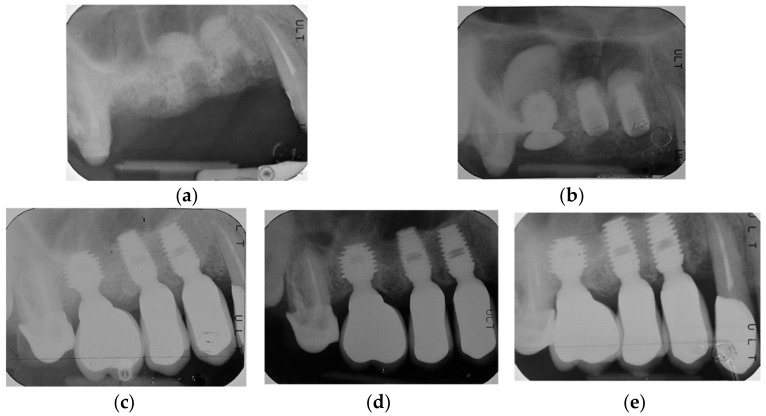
Single implants placed in 1.4, 1.5, and 1.6 sites (4.5 × 8 mm, 4.5 × 8 mm and 5 × 6 mm): (a) pre-operative radiograph before implant placement; (b) radiograph obtained at time of placement; see implant at 1.6 site with sinus lift temporary abutment designed to prevent displacement of the implant into the sinus, also see augmented sinus floor (1.4 sinus lift = 3.8 mm; 1.5 sinus lift = 2 mm; 1.6 sinus lift = 8.5 mm); (c) radiograph obtained at time of loading; (d) radiograph obtained at 3-year follow-up; (e) radiograph obtained at 5-year follow-up. See graft resorption after 3 years and stable bone levels with minimal changes between 3-year (1.4 RCBH = 7.5 mm; 1.4 sinus lift = 2.55 mm; 1.5 RCBH = 6.45 mm; 1.5 sinus lift = 0.5 mm; 1.6 RCBH = 7.1 mm; 1.6 sinus lift = 4.5 mm) and 5-year follow-up (1.4 RCBH = 7.12 mm; 1.4 sinus lift = 2.17 mm; 1.5 RCBH = 6.17 mm; 1.5 sinus lift = 0.47 mm; 1.6 RCBH = 6.45 mm; 1.6 sinus lift = 4.2 mm).
